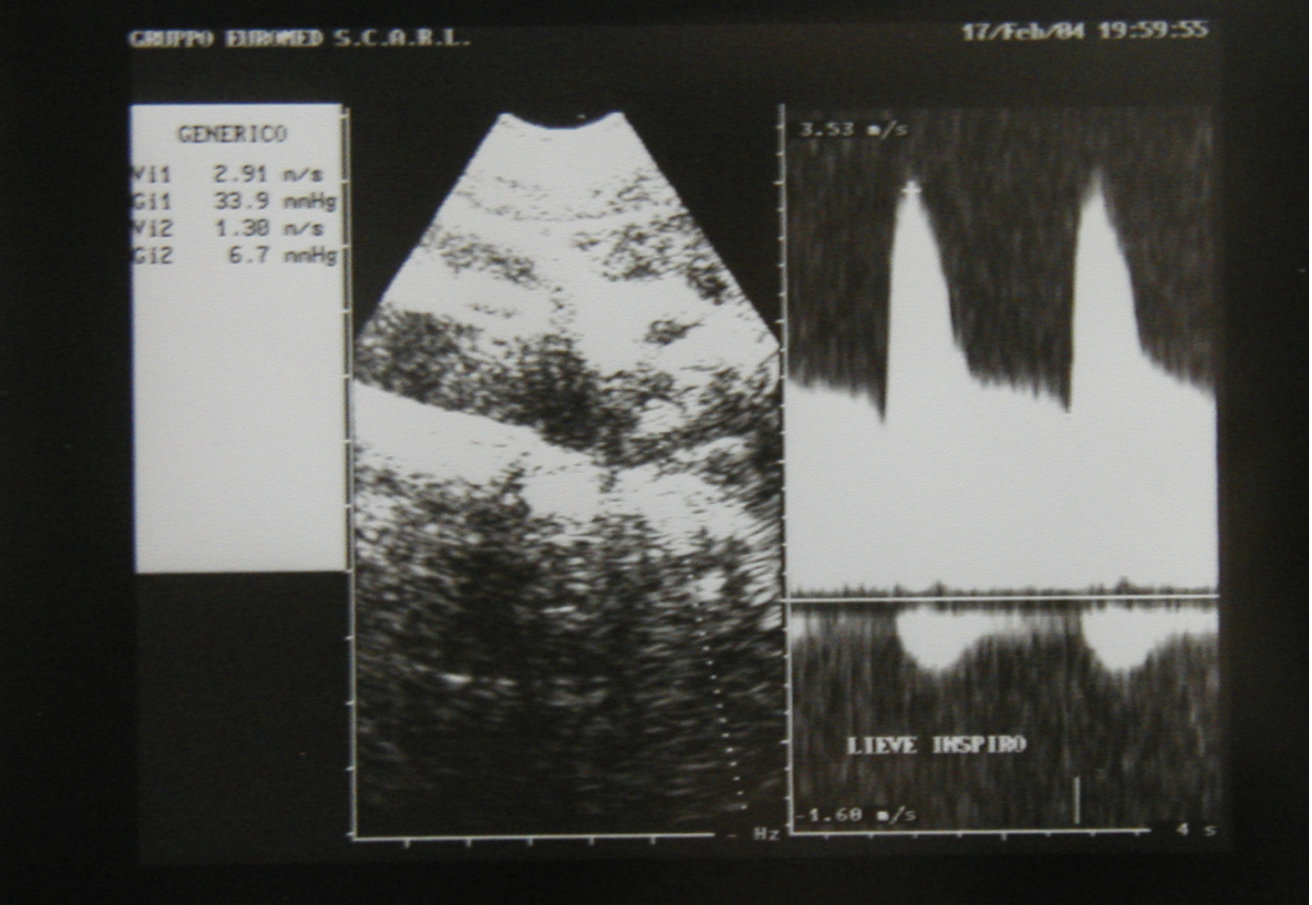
Continuous Doppler of Celiac Artery Stenosis, modeste inspiration.
